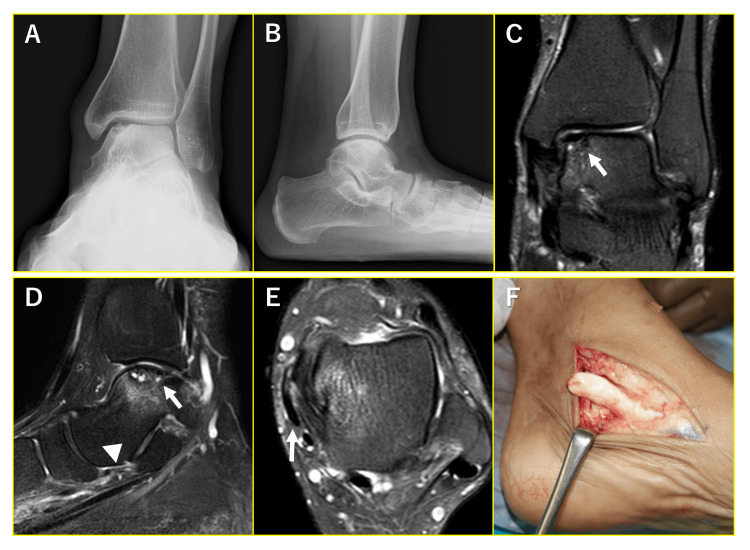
Posterior tibial tendon (PTT) rupture after the fixation of the osteochondral fragment. (A, B) Preoperative radiograms showing medial osteochondral lesion of the talus (OLT) and flatfoot deformity. (C, D) Preoperative MRI (coronal and sagittal images). Arrows indicate OLT and the arrow head indicates the spring ligament with thinning and alteration of signal intensity. (E) Preoperative MRI (axial image). The arrow indicates flattening of PTT. (F) Intraoperative findings of PTT rupture at seven months after OLT surgery.
